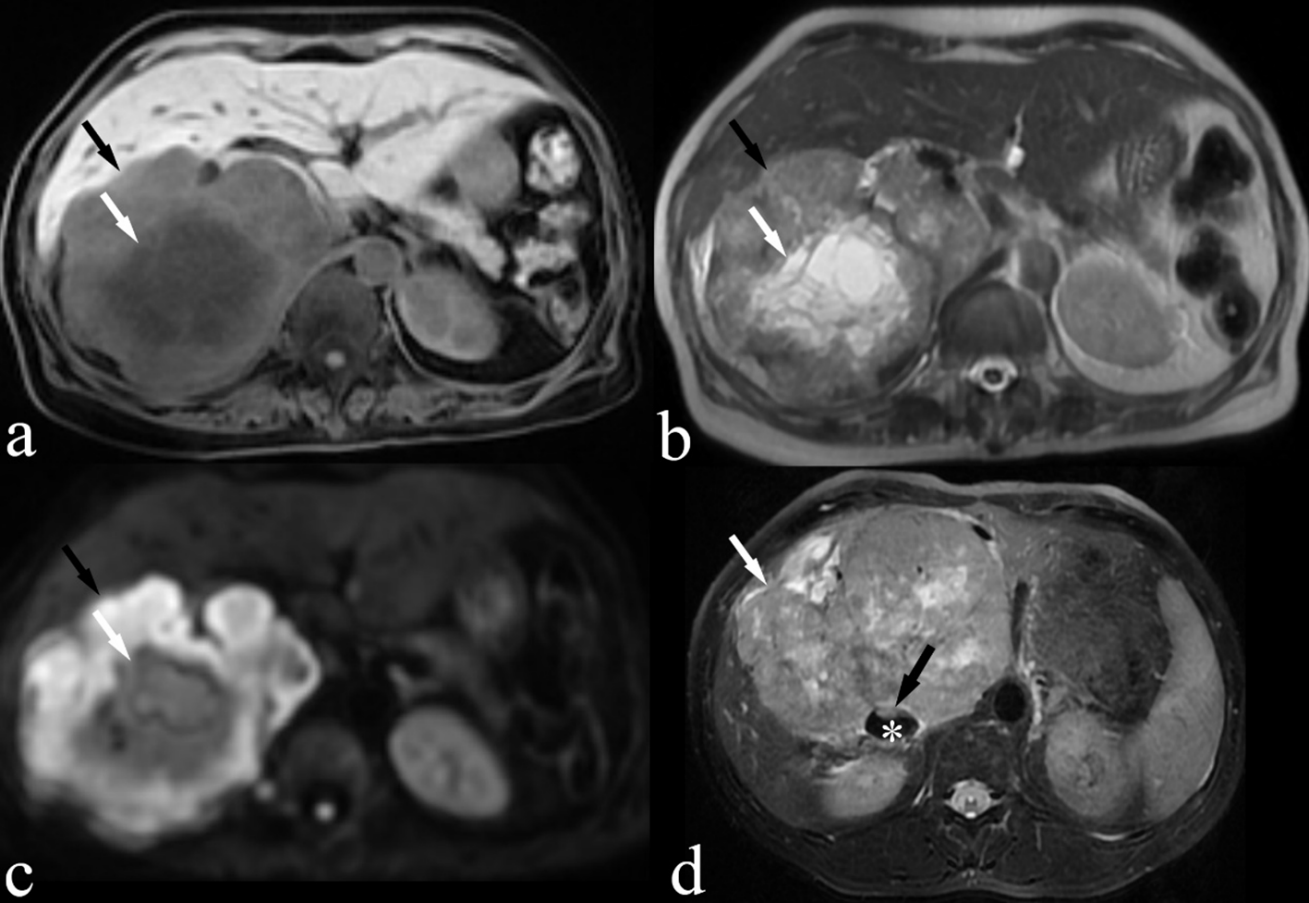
MRI images of a 66-year-old woman (case 3, panels A–C) and a 39-year-old male patient (case 9, panel D) are shown. (A) Fat-suppressed T1-weighted image demonstrating an isointense IVC LMS (black arrow) with focal areas of hypointense suggesting necrosis (white arrow). (B) T2-weighted image demonstrating a slightly hyperintense IVC LMS with focal areas of hyperintense suggesting necrosis (white arrow) (C) Diffusion-weighted image demonstrating hyperintense IVC LMS (black arrow). (D) Fat-suppressed T2-weighted image demonstrating a slightly hyperintense IVC LMS (white arrow) with a nodular protrusion (black arrow) within the IVC (*).
